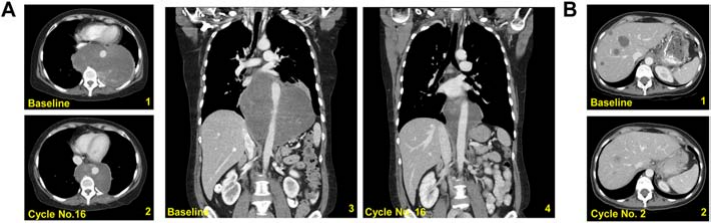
CT scans of patients who responded to trabectedin. a Patient with recurrent metastatic grade II myxoid liposarcoma encasing the aorta. Paired axial (1,2) and coronal (3,4) cuts showing a partial response after 16 cycles of trabectedin. b Patient with recurrent poorly differentiated leiomyosarcoma that metastasized to the liver. Paired axial (1,2) cuts showing a partial response after 2 cycles of trabectedin
